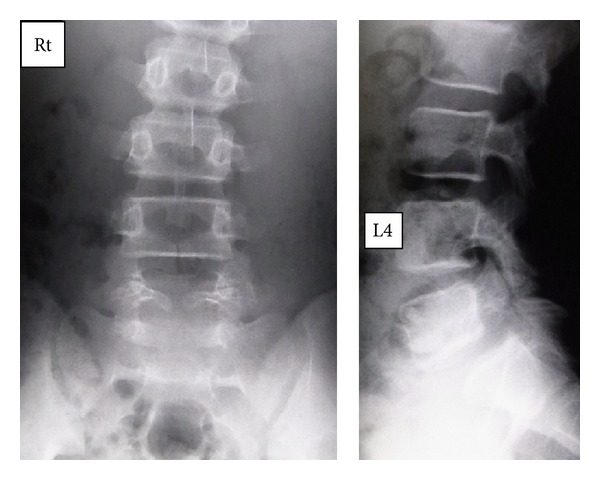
Plain radiographs of Case 1. Spina bifida occulta (SBO) and L5 isthmic olisthesis are obvious.
